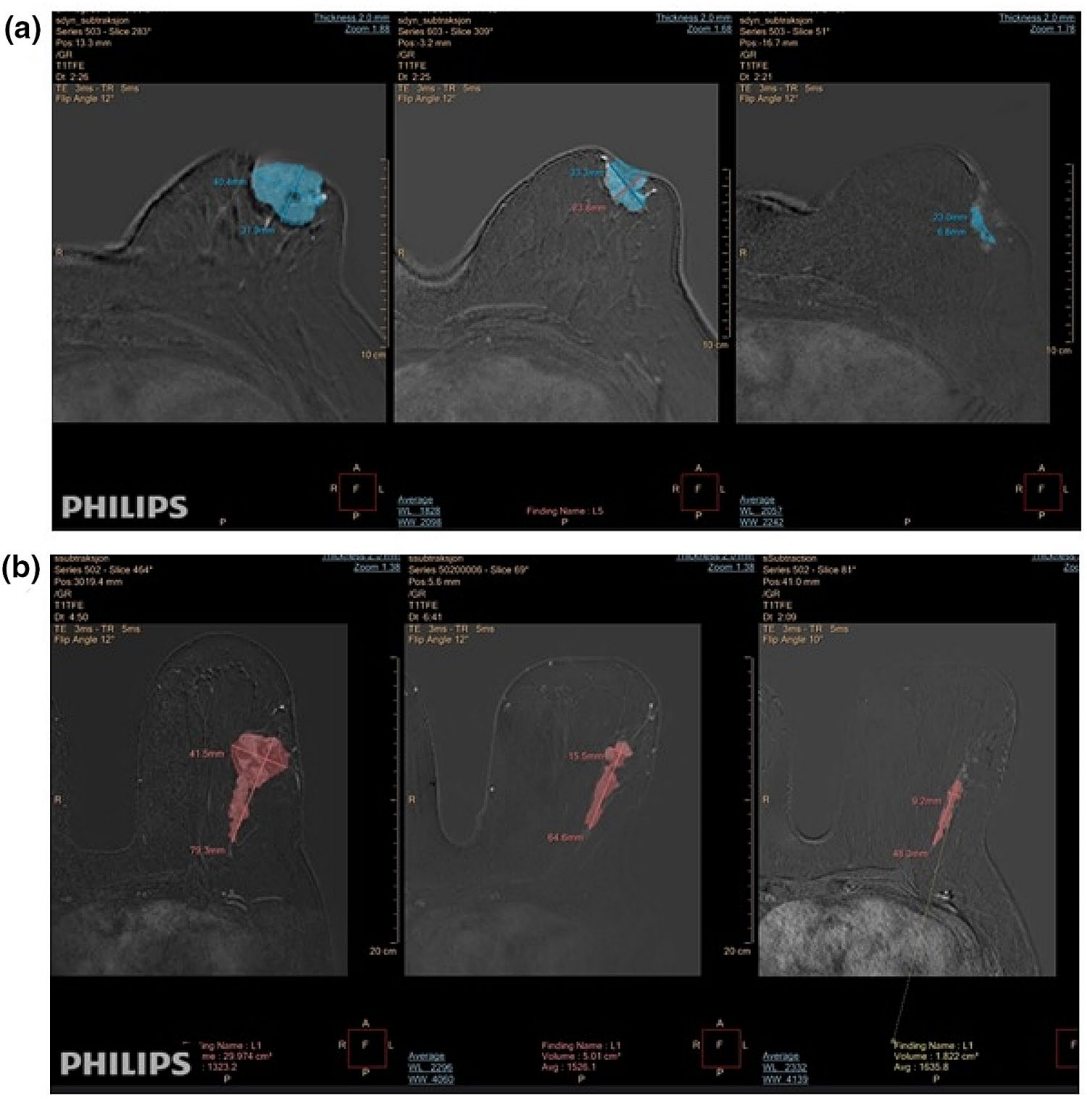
a, b Two different patients’ magnetic resonance imaging examinations demonstrating response to treatment and residual tumour sizes. Manual assessment of tumour longest diameter using the tumour tracking system incorporated in Philips IntelliSpace Portal program. Description of the time points from left to right: at baseline, between regimens (after 2 months of treatment) and presurgery (after 4 months of treatment)
